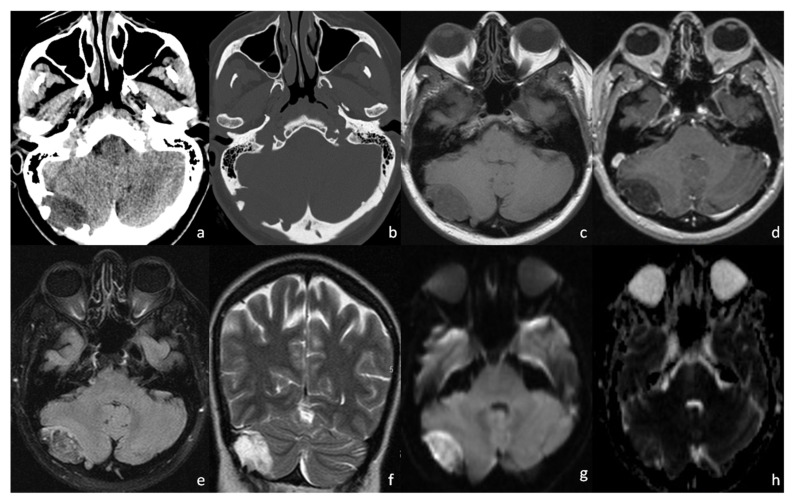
Case 3: CT scan (a,b) showing a thecal-centered, inhomogenously hypodense mass causing bone remodeling and focal areas of bone erosion. The 1,5 T MRI (c–h) of the lesion, which appears unevenly hypointense on T1WI (c), and hyperintense on T2WI (f). There is incomplete fat saturation on FLAIR (e) and on post-contrast 3D T1 MPRAGE (d); and poor restricted diffusion on DWI/ADC (g,h). On 3D T1 MPRAGE (d) subtle contrast enhancement of the capsule and some thin internal septa may be appreciated.
